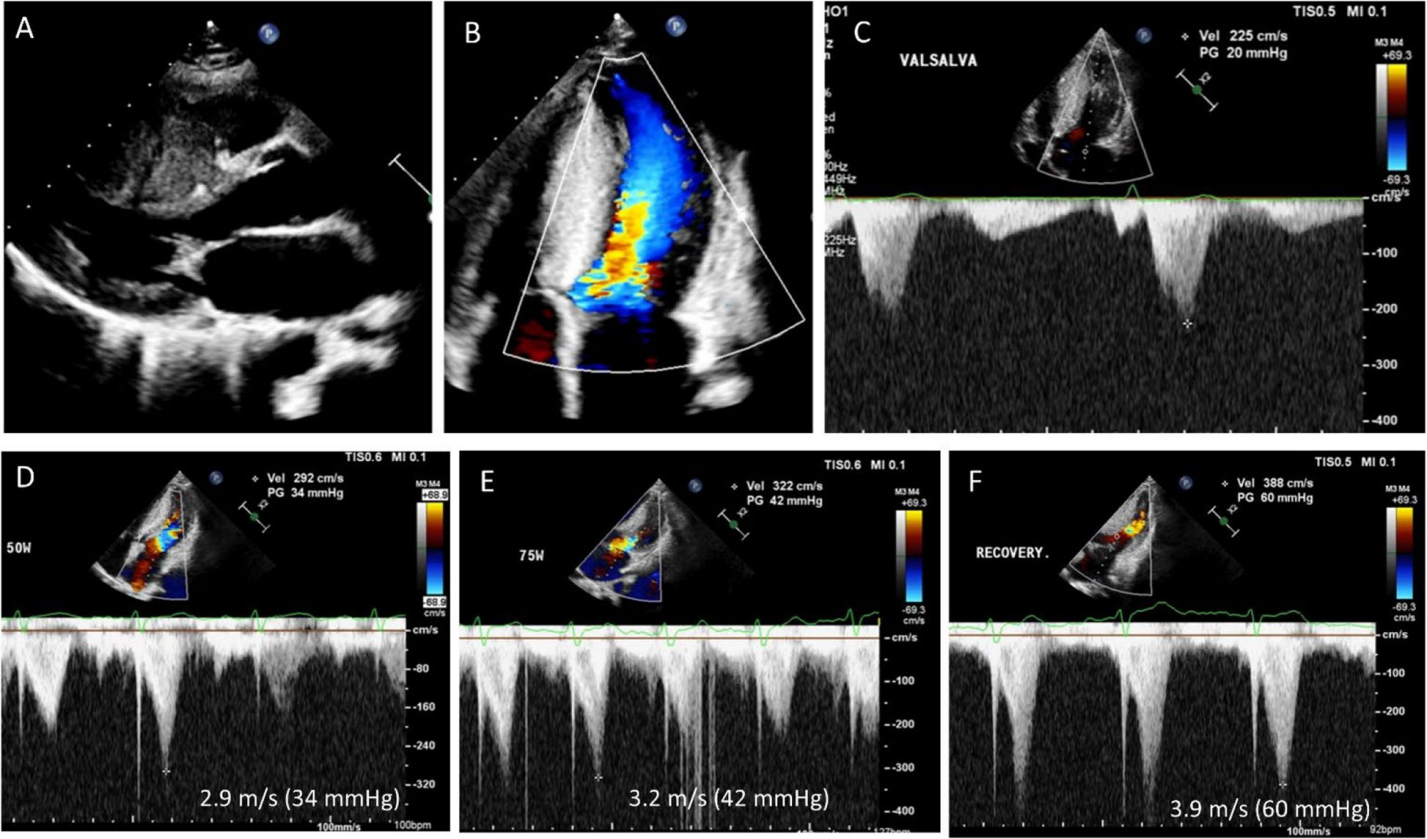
A representative case showing significant left ventricular outflow tract obstruction immediately after exercise. A 32-year-old woman developed repeated episodes of syncope after running short distances. The syncope usually developed after the exercise; during exercise, she did not experience any discomfort. (A–C) Resting echocardiography showed typical asymmetrical septal hypertrophy without systolic anterior motion of the mitral valve or left ventricular outflow tract obstruction at rest and with the Valsalva maneuver. (D, E) During a semi-supine bicycle exercise, significant left ventricular outflow tract obstruction was absent; (F) however, immediately after exercise, a delayed peak velocity of 3.9 m/sec (60 mmHg) was recorded
